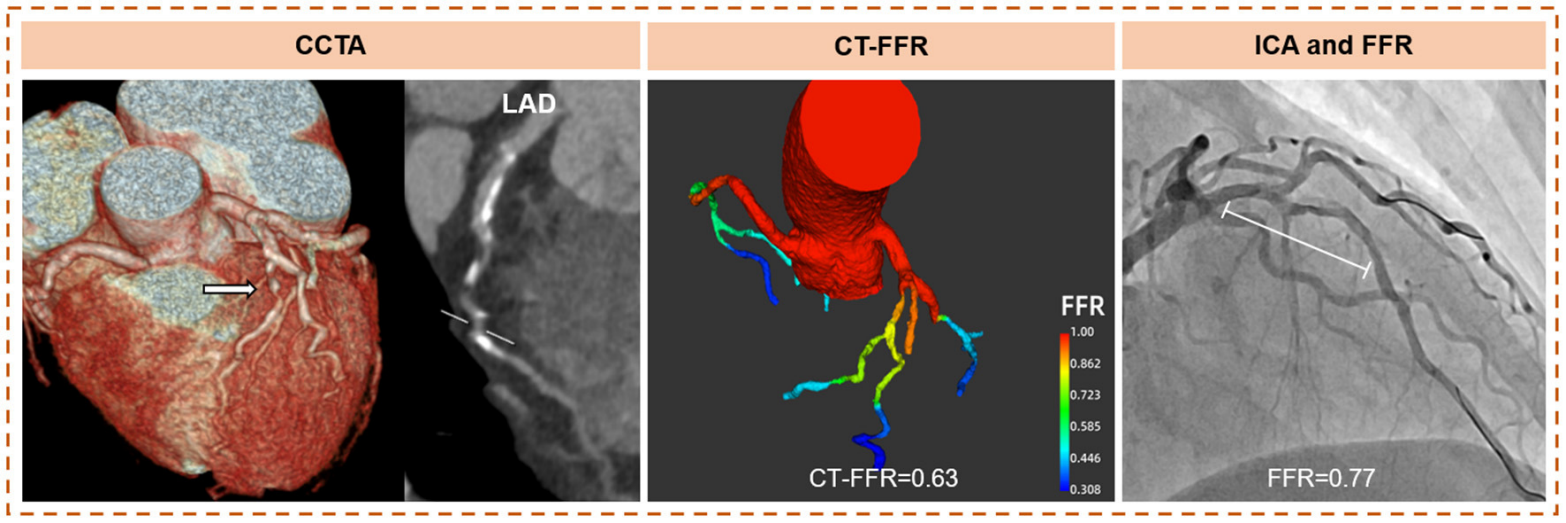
Case of CT-FFR estimating coronary lesions with severe calcification. Example of a 66-year-old female with stable chest pain. The CAC scores of LAD, LCX, and RCA were 599.6, 216.4, and 716.2. CCTA showed multiple calcified and mixed plaques causing more than 70% stenosis in the proximal and mid LAD. CT-FFR demonstrated functional ischemia (CT-FFR = 0.63) caused by LAD stenosis, and confirmed by FFR (FFR = 0.77). The deviation of CT-FFR was 0.14 in the situation of severe coronary calcification. With the widely-accepted threshold of ischemia ( ≤ 0.8), CT-FFR made a correct dichotomous diagnosis. LAD, left anterior descending coronary artery; LCX, left circumflex artery; RCA, right coronary artery; CT-FFR, computed tomography derived fractional flow reserve; FFR, fractional flow reserve; CAC, coronary artery calcium; CTA, computed tomography angiography.
